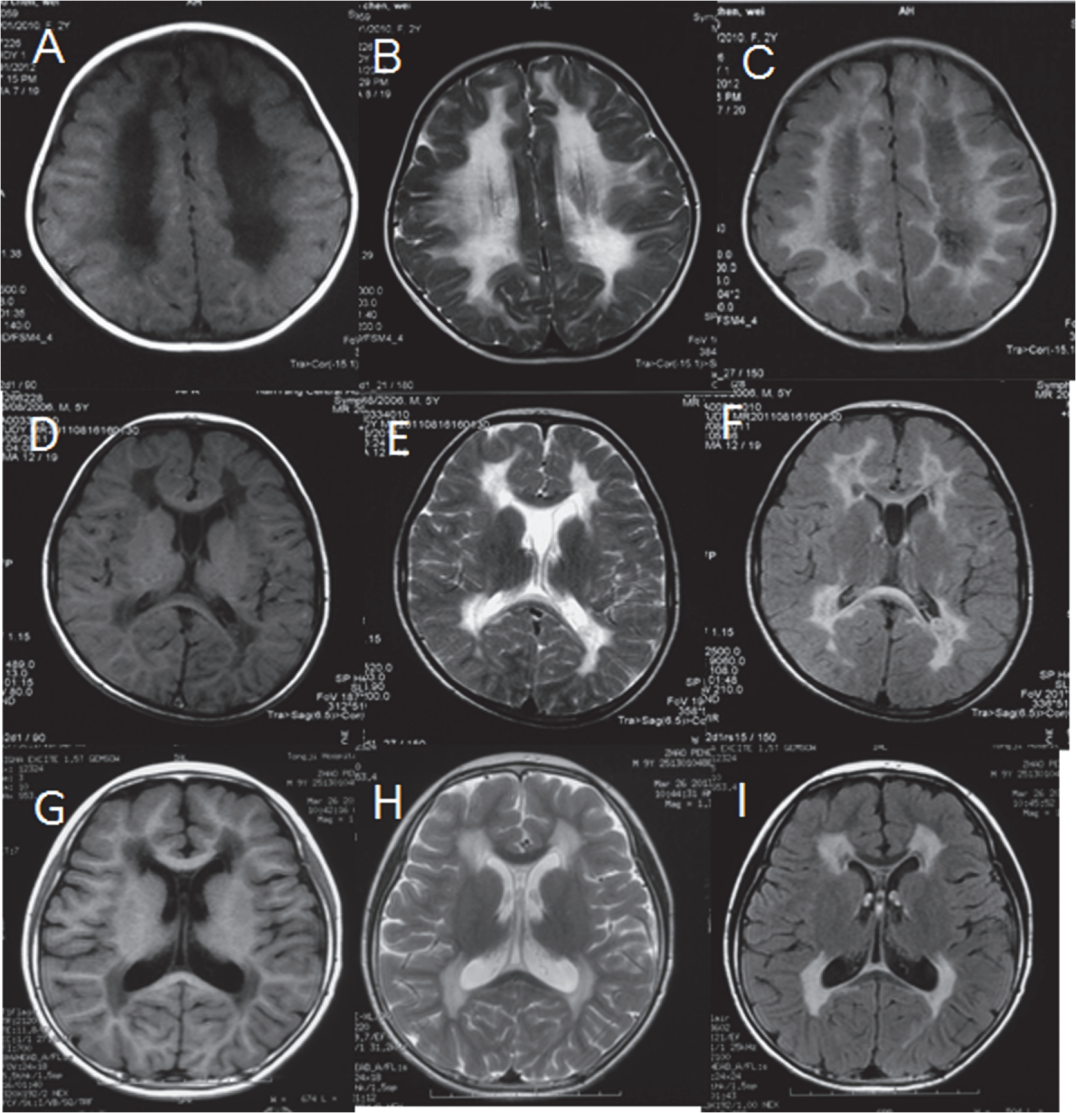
Brain MRI in VWM children. MRI from an infantile case (A, B, C), an early childhood case (D, E, F) and a juvenile case (G, H, I) respectively. In infantile and early childhood case, diffuse and symmetrical low signal in T1 (A, D), high signal in T2 (B, E) and Flair (C, F) images were shown in cerebral white matter, with part of white matter becomes CSF-like signal (low signal in Flair images). The white matter involvement in the juvenile case is milder, with only periventricular white matter and inner rim of corpus callosum involved and without CSF-like changes.
